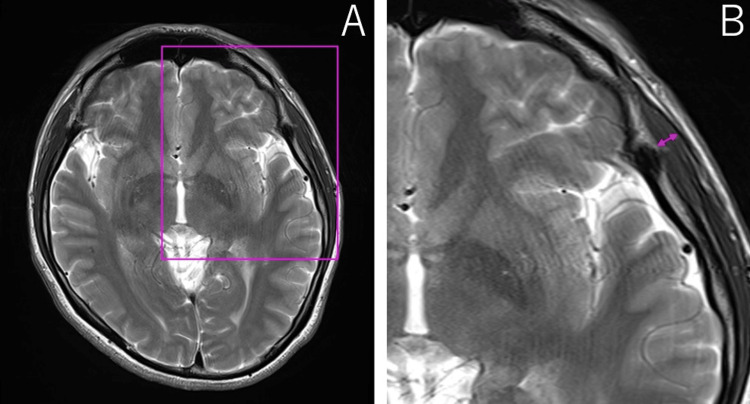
TMT assessment represented on cranial T2-weighted contrast-enhanced MRIs (A) Axial T2-weighted image obtained at the level of the orbital apex. (B) The supraorbital fissure and orbital apex were used as anatomical landmarks. The Sylvian fissure was identified as a reference point for the anterior-posterior orientation. TMT was measured bilaterally, perpendicular to the long axis of the muscle. TMT, temporal muscle thickness; MRI, magnetic resonance imaging
